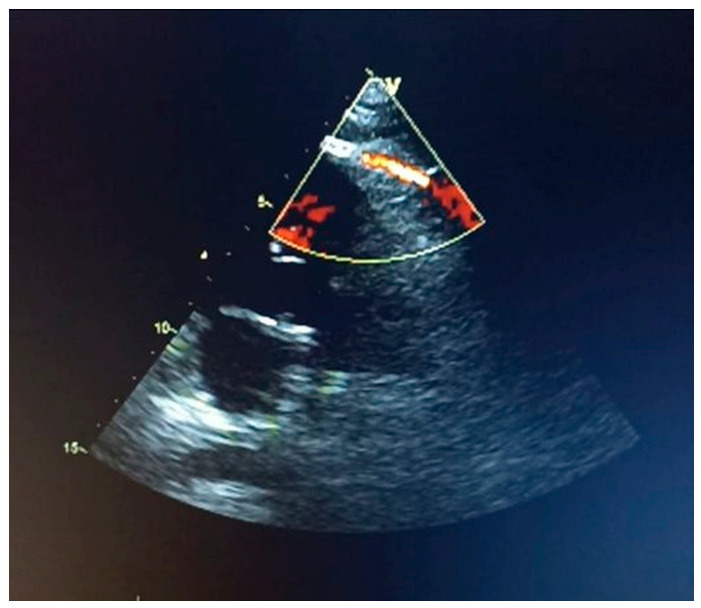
Transthoracic Doppler echocardiography. Color Doppler visualization of mid-diastolic LAD.
